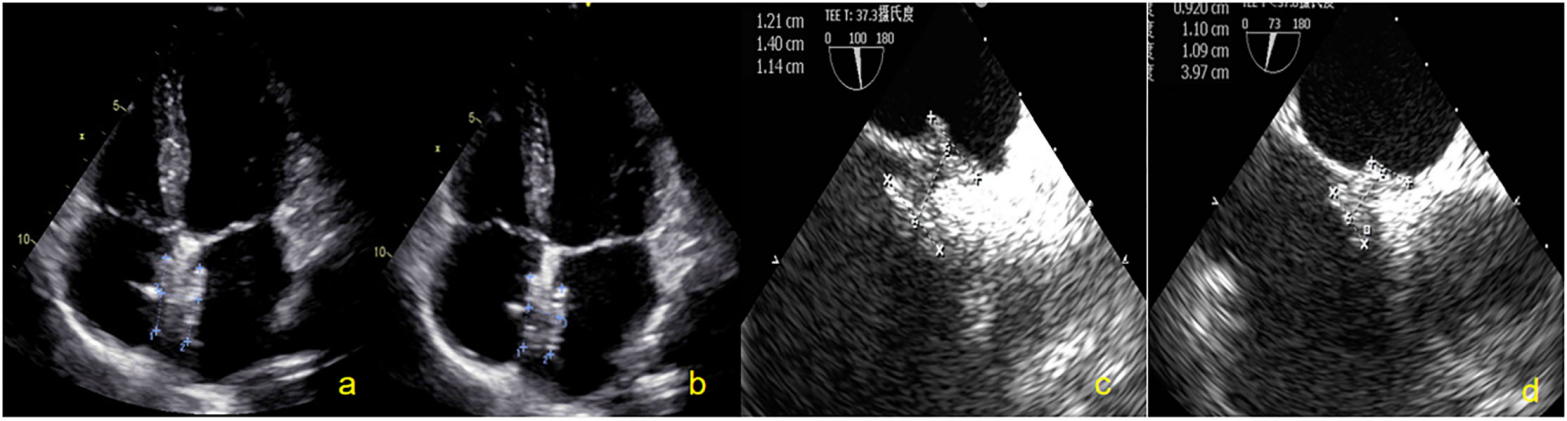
Subjects were followed up by TTE and TEE. (a) Two-dimensional TTE displaying the size of the NBO at 2 days. (b) Two-dimensional TTE displaying the size of the NBO at 3 months. (c) Two-dimensional TEE showing the size of the NBO at 6 months. (d) Two-dimensional TEE showing the size of the NBO at 12 months. NBO, Novel biodegradable occlude; TTE, Transthoracic echocardiography; TEE, Transesophageal echocardiography.
